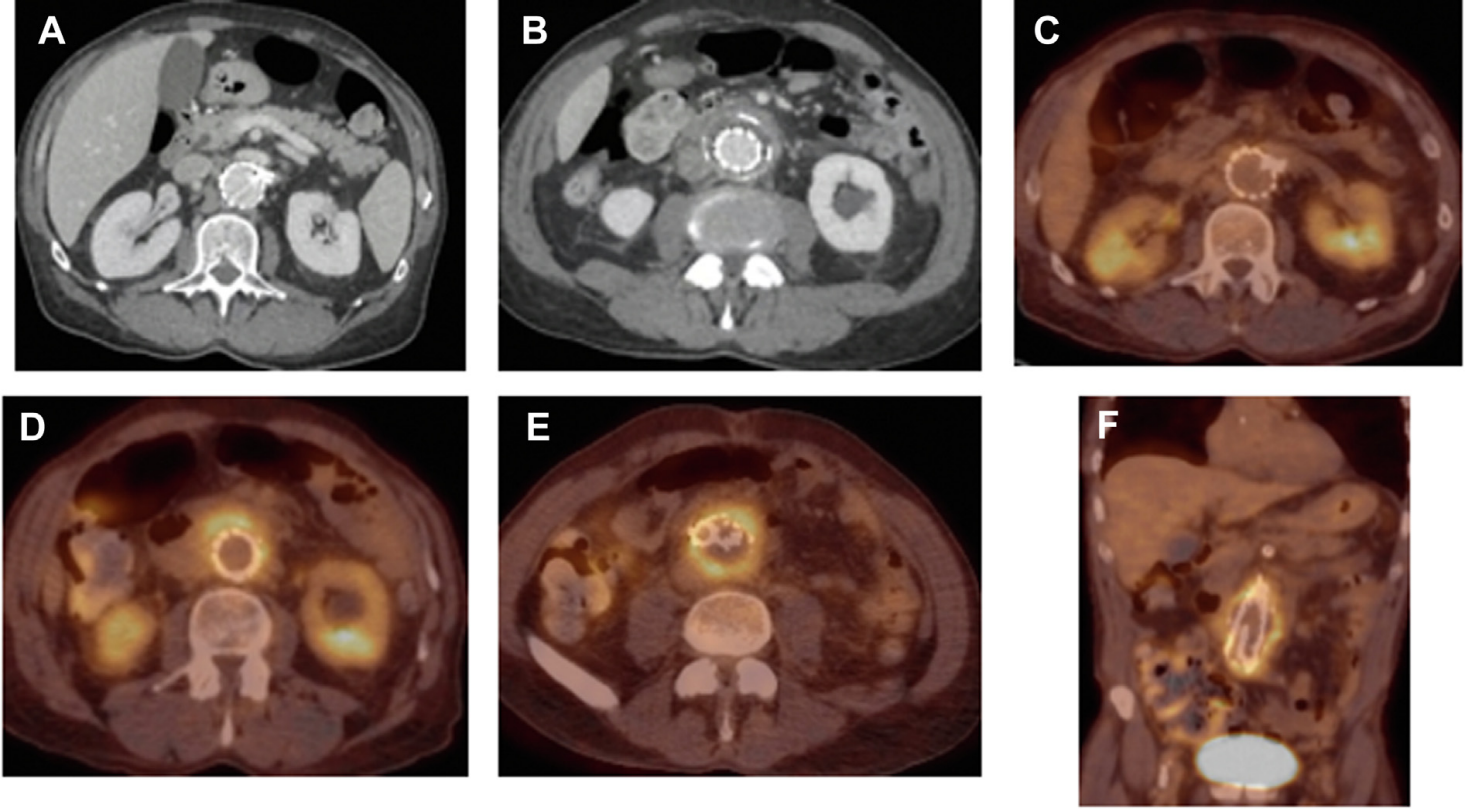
Preoperative computed tomography angiography and positron emission tomography (PET). Note the well-incorporated graft in the paravisceral segment (A) and thickening and/or inflammatory changes of the infrarenal aorta concerning for infection or aortitis (B). Similarly, note that the paravisceral aorta is free of any uptake on the preoperative PET scan in the paravisceral aorta (C) but the significant uptake in the infrarenal aorta was limited to the aortic sac, sparing the endograft itself (D-F).
